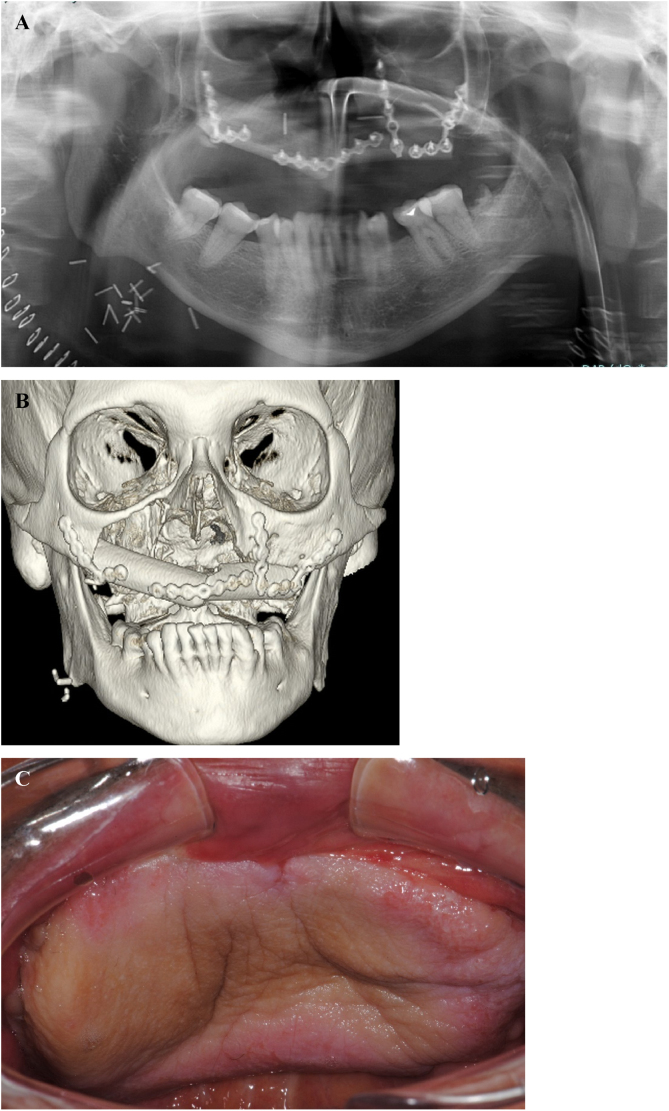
Total maxillectomy and reconstruction with fibula free flap: orthopantomography (A) and 3D-CT images (B); clinical picture at follow-up examination, 6-months after surgical treatment (C).
